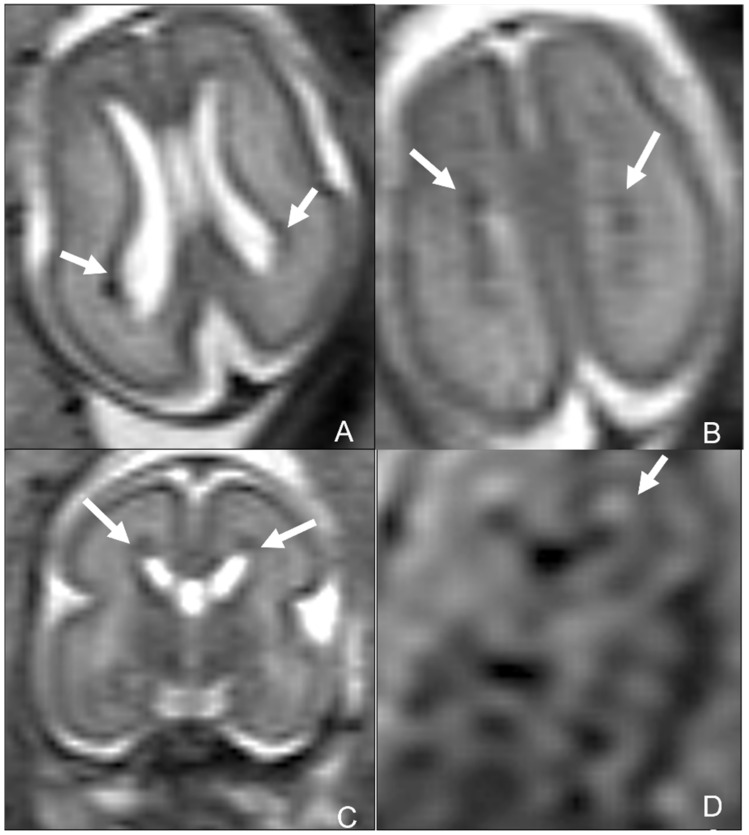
Fetus of 23 weeks of gestation age affected by parvovirus and presenting with anemia, cardiac failure, hydrops, and venous congestion. Fetal MRI shows hemorrhagic venous infarctions in deep medullary veins (white arrows in (A–D)).
